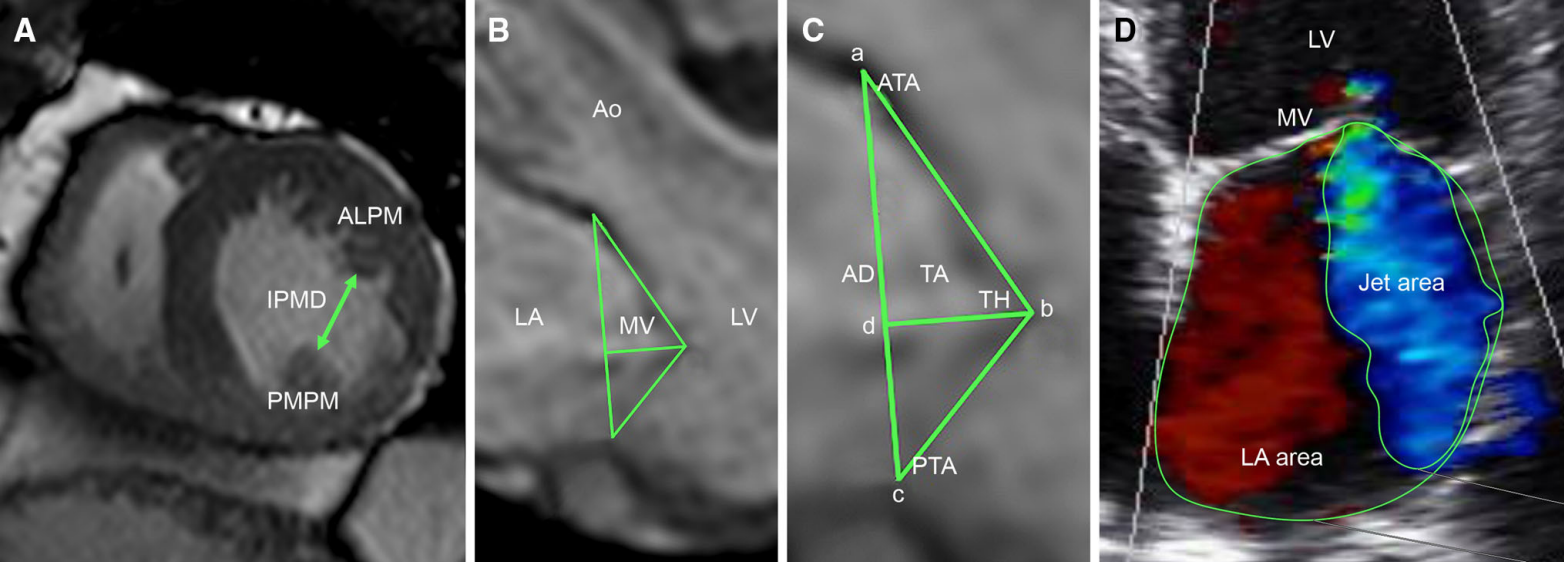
Evaluation of interpapillary muscle distance, mitral valve geometry, and CIMR severity. A evaluation of interpapillary muscle distance measured with cardiac MRI in the short-axis view (end-systolic). B, C Evaluation of mitral valve geometry with cardiac MRI in the 3-chamber view (mid-systolic); mitral annular diameter (*a*–*c*), tethering height (*d*–*b*), tethering area (area enclosed by *a*–*b*–*c*), posterior tethering angle (angle between *c*–*a* and *c*–*b*), anterior tethering angle (angle between *a*–*c* and *a*–*b*). D Evaluation of CIMR severity with TTE in the apical four-chamber view (mid-systolic) (jet area/LA area). *AD* annular diameter, *ALPM* anterolateral papillary muscle, *Ao* aorta, *ATA* anterior tethering angle, *CIMR* chronic ischemic mitral regurgitation, *IPMD* interpapillary muscle distance, *LA* left atrium, *LV* left ventricle, *MV* mitral valve, *PMPM* posteromedian papillary muscle, *PTA* posterior tethering angle, *TA* tethering area, *TH* tethering height
